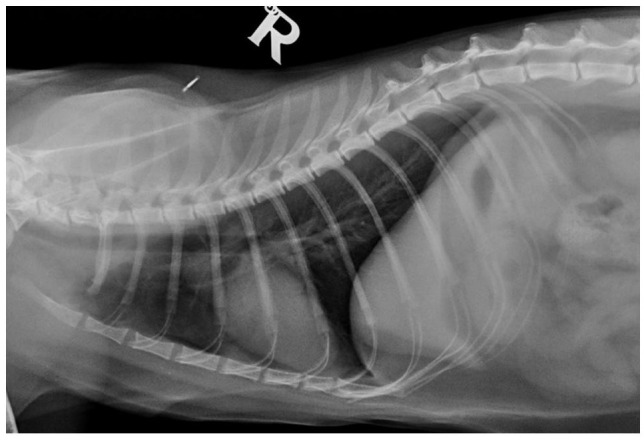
Right lateral thoracic radiograph demonstrating a caudodorsal interstitial lung pattern
